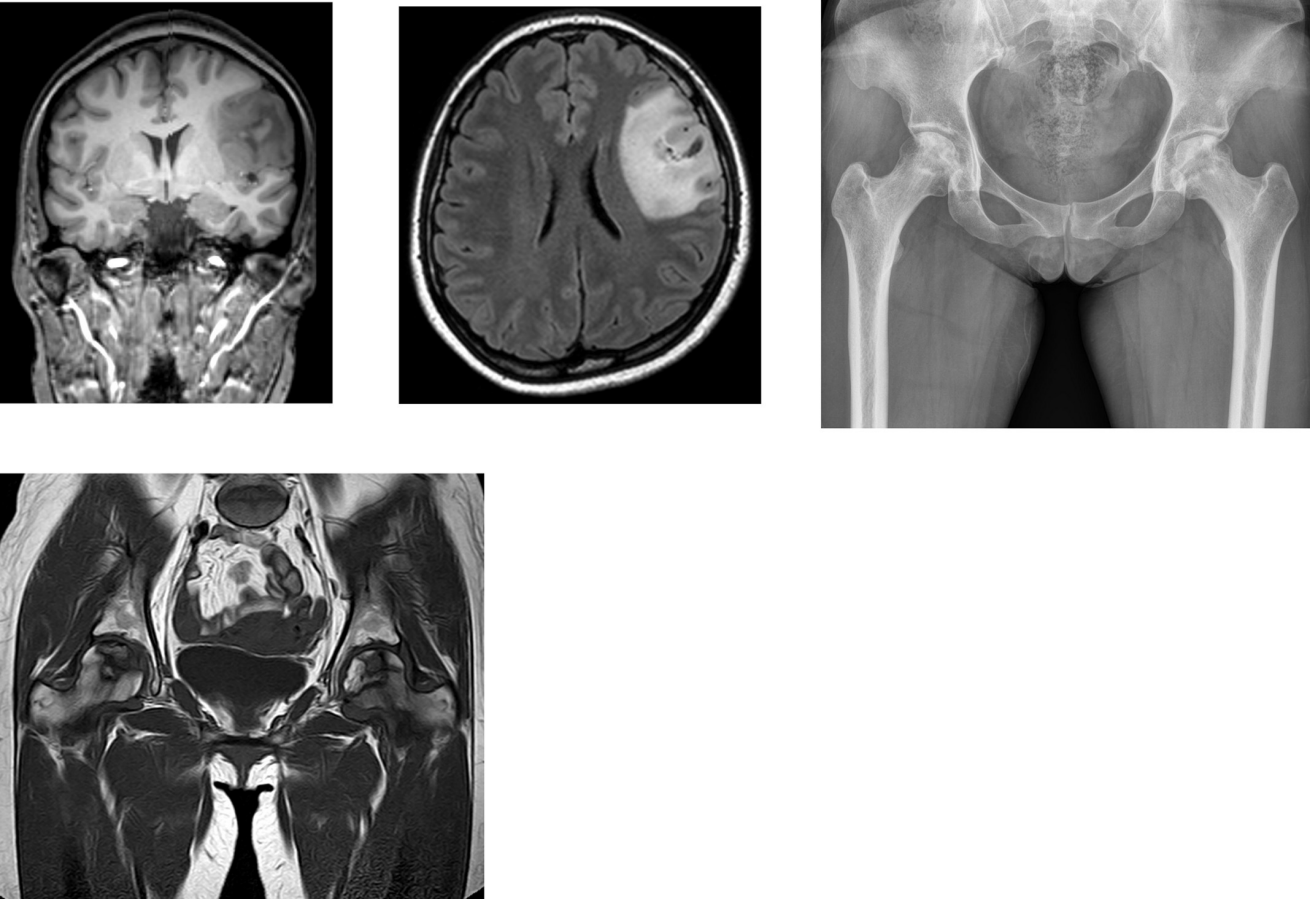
Example of a newly diagnosed osteonecrosis of the femoral head in brain tumor patient. (A, B) Brain magnetic resonance imaging (MRI) of a 25-year old woman who had generalized tonic–clonic seizures, revealed a tumor in the left frontal lobe. She was diagnosed with pathology-confirmed diffuse astrocytoma by navigation-guided biopsy. She received a total of 105 mg dexamethasone and underwent brain radiotherapy. (C, D) The patient suffered from hip pain 2 years after the astrocytoma diagnosis. Anteroposterior hip radiograph and MRI show osteonecrosis of the femoral head involving both hips.
